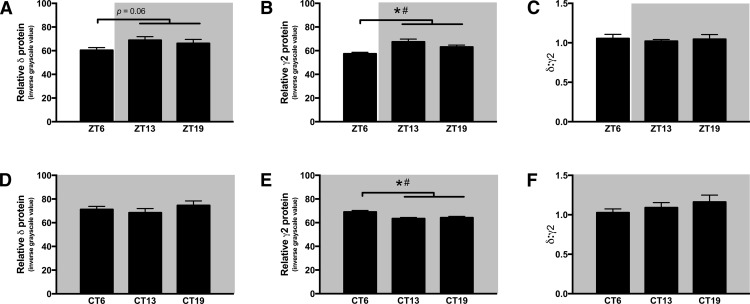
Rhythmic GABA_A_R subunit protein-IR in the SCN. Protein-IR of the synaptic GABA_A_γ2 receptor varied by circadian phase and CT in a 14:10 LD cycle (*B*), with the highest amount of protein-IR during the night (ZT13 and ZT19; active phase). However, after 10 d of free running in DD conditions, the rhythm of synaptic GABA_A_γ2 protein-IR was inverted with highest levels of protein-IR found during the subjective day (CT6, inactive phase; *E*). The extrasynaptic GABA_A_δ receptor protein-IR did not vary by time of day or phase in either LD or DD conditions (*A*, *D*). The ratio of δ-IR:γ2-IR did not significantly vary in the whole SCN in either LD or DD (*C*, *F*). p* ≤ 0.05 active versus inactive phase; #*p* ≤ 0.05 ANOVA. Statistics in Table 3.
